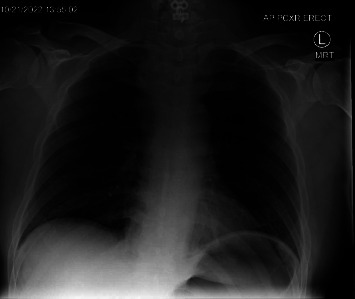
Initial cervical fusion postoperative chest radiograph.
